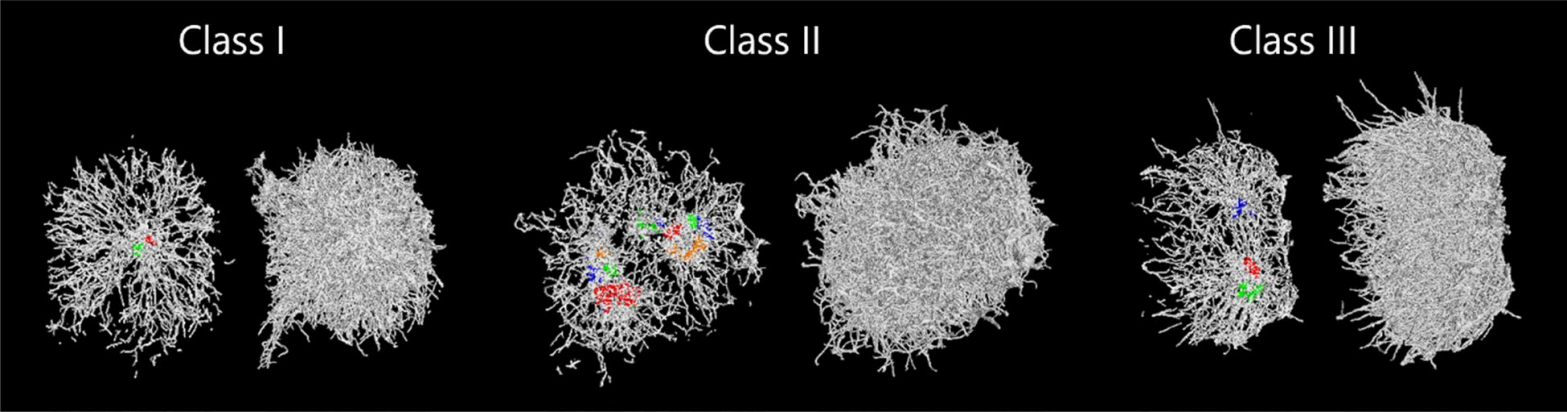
Pellet classification based on inner structure. The images obtained from SR-µ-CT measurements depict a 25 µm-thick cross-section through the centre of mass of the pellet (left) created by 3D rendering of the hyphae and talc (if applied). Spore clusters were calculated by a density-based clustering algorithm ("dbscan", MATLAB) with a minimum of 100 spores. For enhanced visibility, the calculated spore clusters of the presented cross-section are shown in different colours and the spores are enlarged by a factor of 9. Additionally, the outer morphology of each pellet type is presented for comparison (right). The images were created using VGSTUDIO MAX (version 3.2; Volume Graphics GmbH)
